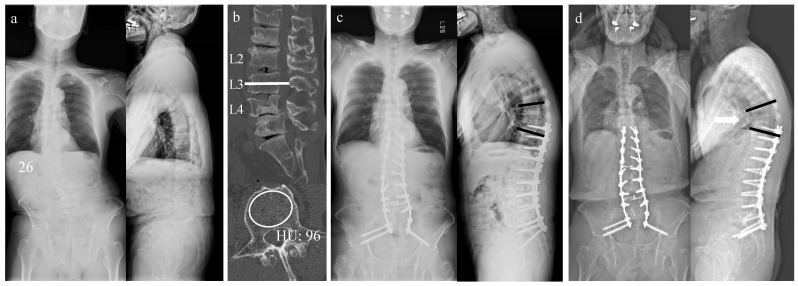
Representative case: (a) Preoperative standing whole-spine anteroposterior and lateral views. (b) Preoperative computed tomography views. (c) Immediate postoperative standing sagittal and lateral views. (d) Two years postoperative standing sagittal and lateral views. Black line means proximal junctional angle. The white arrows indicate the occurrence of PJK.HU: Hounsfield unit; PJK: proximal junctional kyphosis.
